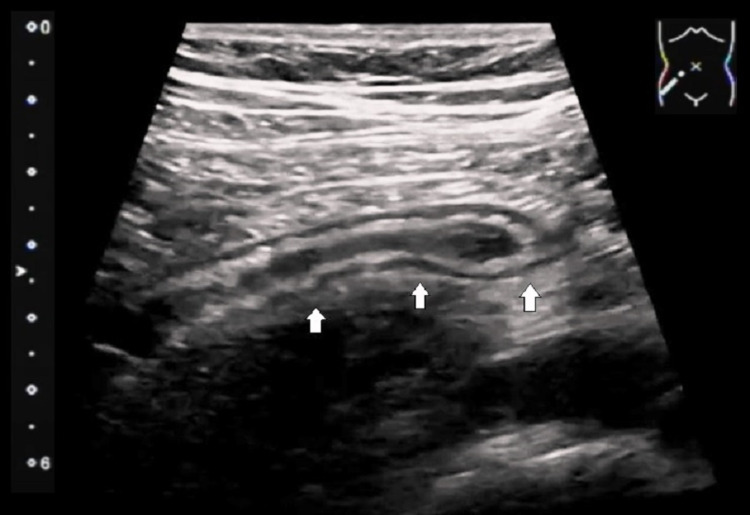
Longitudinal ultrasound image of the right lower quadrant An inflamed appendix (white arrows) in the right lower quadrant giving the shape of a dilated blind-ended tubular structure with mural thickening and mild periappendiceal fluid
